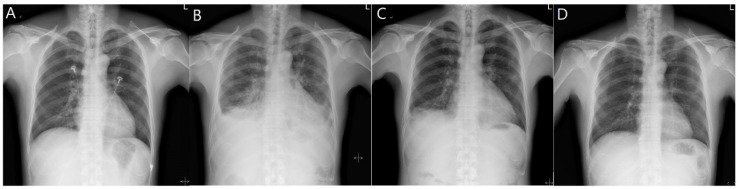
Changes in plain chest radiography (PA) with the clinical course. (A) No active disease at the time of admission. (B) Deterioration during hospitalization (6 days after admission); pneumonia or pulmonary edema; bilateral pleural effusion or thickening. (C) Improvement in pulmonary edema 13 days after admission. (D) No active disease at the time of outpatient follow-up (7 days after discharge).
